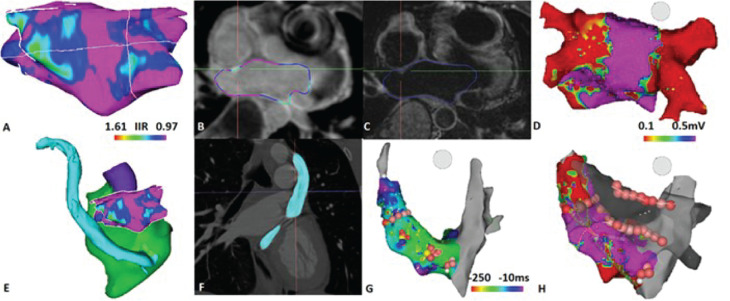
Examples of the utility of image integration for AF ablation. A: CMR-segmented left atrial shell [posteroanterior (PA) view]. The image intensity ratio (IIR) was used to define atrial scar (IIR > 1.61 correlates with < 0.1 mV; IIR < 0.97 correlates with ; 0.5 mV). The patient underwent prior PVI five months before imaging. B: High-resolution LGE cardiac MRI image (axial view). An atrial scar is noted at the bilateral pulmonary vein antra. C: T2-space cardiac MRI image (axial view). Better identification of the atrial wall of the left atrium is apparent. D: Before ablation, a prior ablation scar at the bilateral PV antra is observable (voltage map, PA view). E: Display of CMR-segmented left atrial scar shell and CT-segmented left-sided SVC. F: Left-sided SVC on CT angiography. G: Non-PV triggers at the ostium of the coronary sinus, proximal coronary sinus, and left-sided SVC (ablation lesions on non-PV triggers, activation map). H: Final ablation lesions on the posterior wall, non-PV triggers, and left-sided SVC isolation (voltage map of left-sided SVC).
